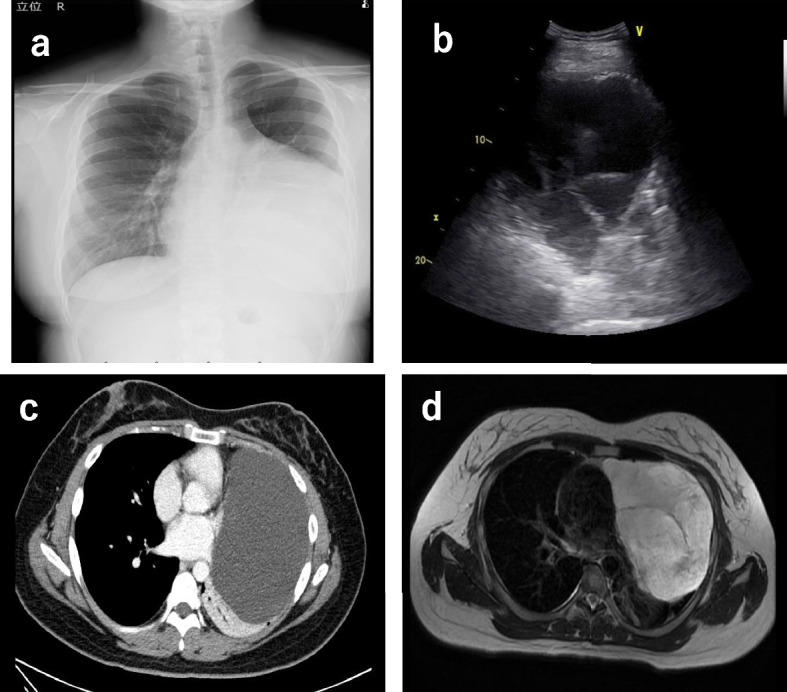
Preoperative imaging examination. a Chest X-ray revealed a huge tumor in the left lung field. b Body surface echocardiography revealed a multifocal cystic tumor. c Contrast-enhanced CT on a close examination indicated a massive cystic lesion 20 cm in size occupying the left thoracic cavity, contiguous with the mediastinum. d Contrast-enhanced MRI revealed a multifocal cystic tumor with no evidence of invasion into surrounding tissues
